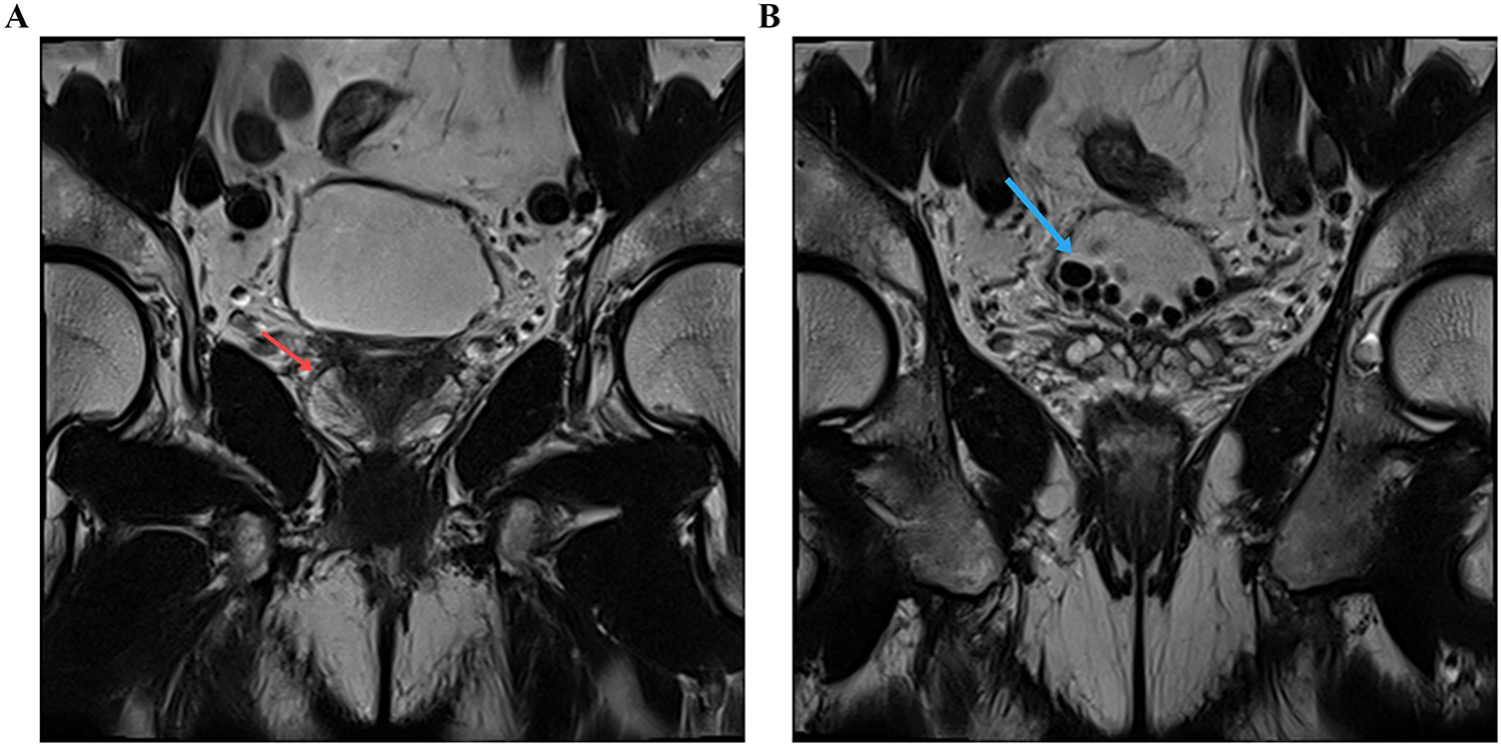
Magnetic resonance imaging revealing the (A) enlarged prostate and (B) bladder stones.
